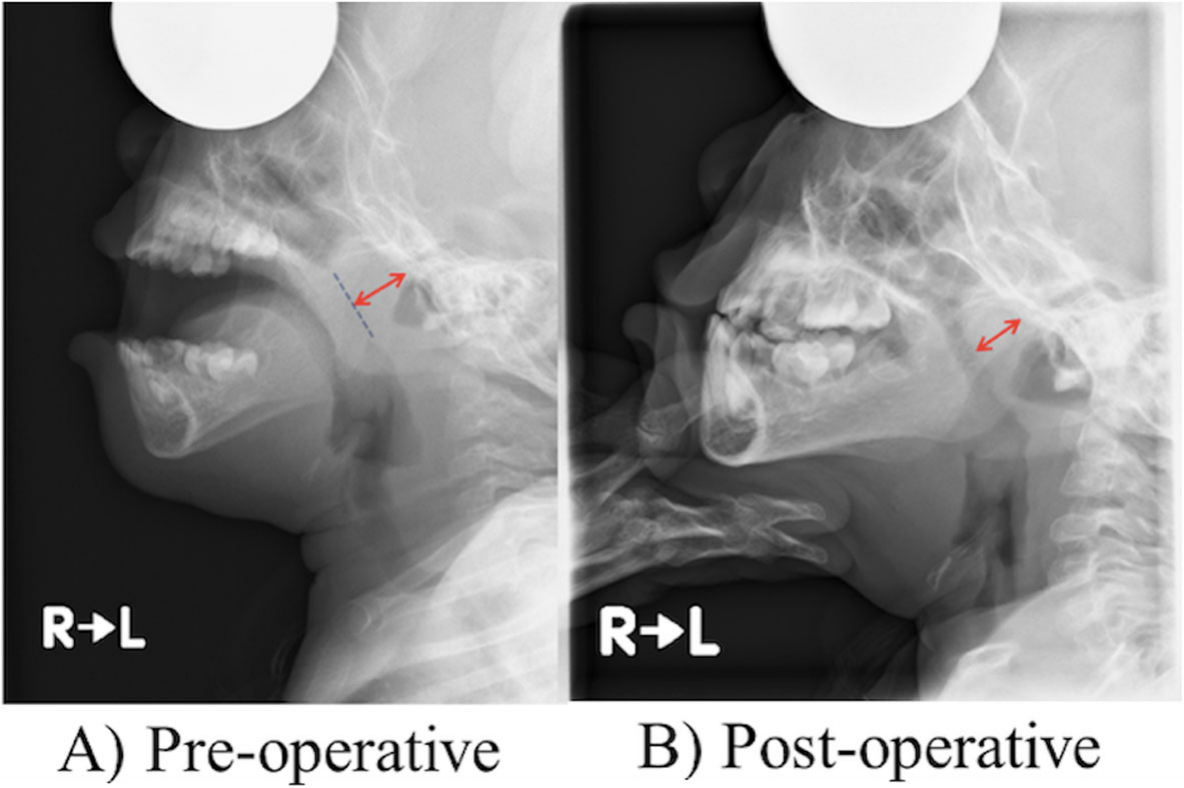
Pre- and postoperative lateral-view radiographs of the nasopharynx. a Preoperative image. b Postoperative image. Bloated adenoids (a: arrow) encroach on and severely obstruct the nasopharyngeal airway (a: dotted line). After tonsillectomy (b: arrow), the nasopharyngeal airway is open, allowing the patient to breathe with the mouth closed
